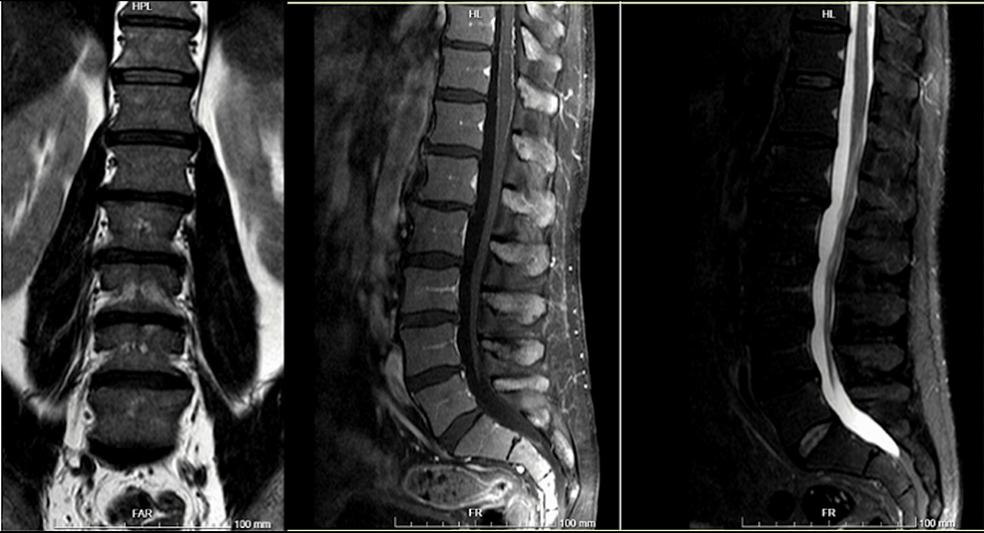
Lumbosacral magnetic resonance imaging (MRI) performed one week after the event
